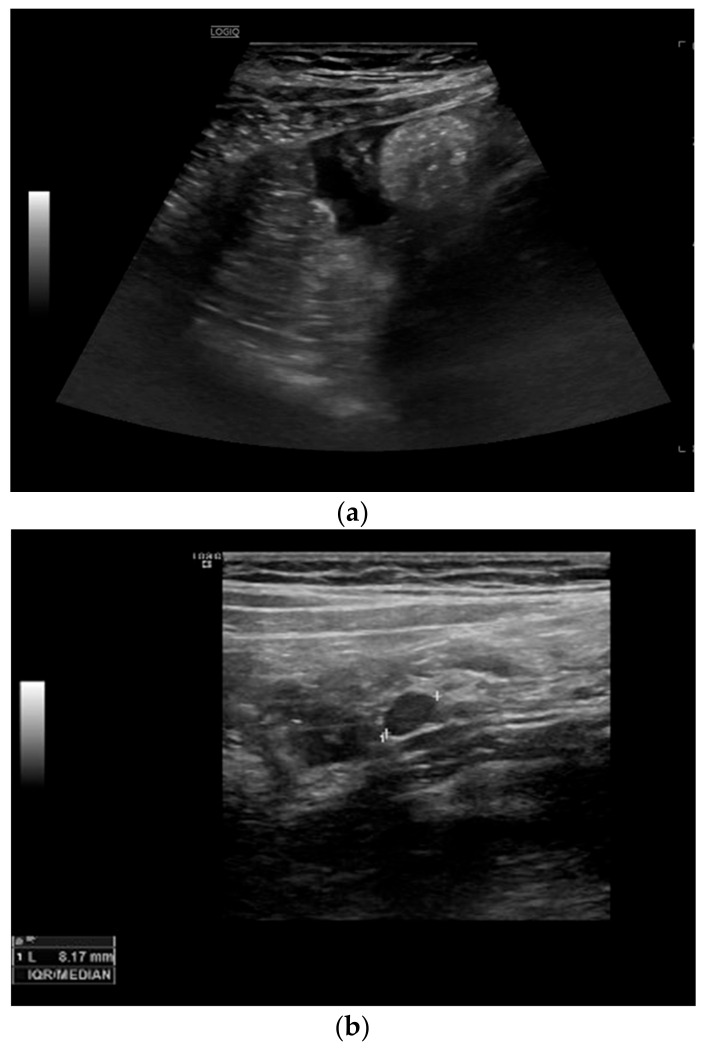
Extraintestinal ultrasonographic findings. (a) Presence of free fluid. (b) Detection of a lymph node and presence of fibro-fatty proliferation (echogenic peri-enteric fat).
